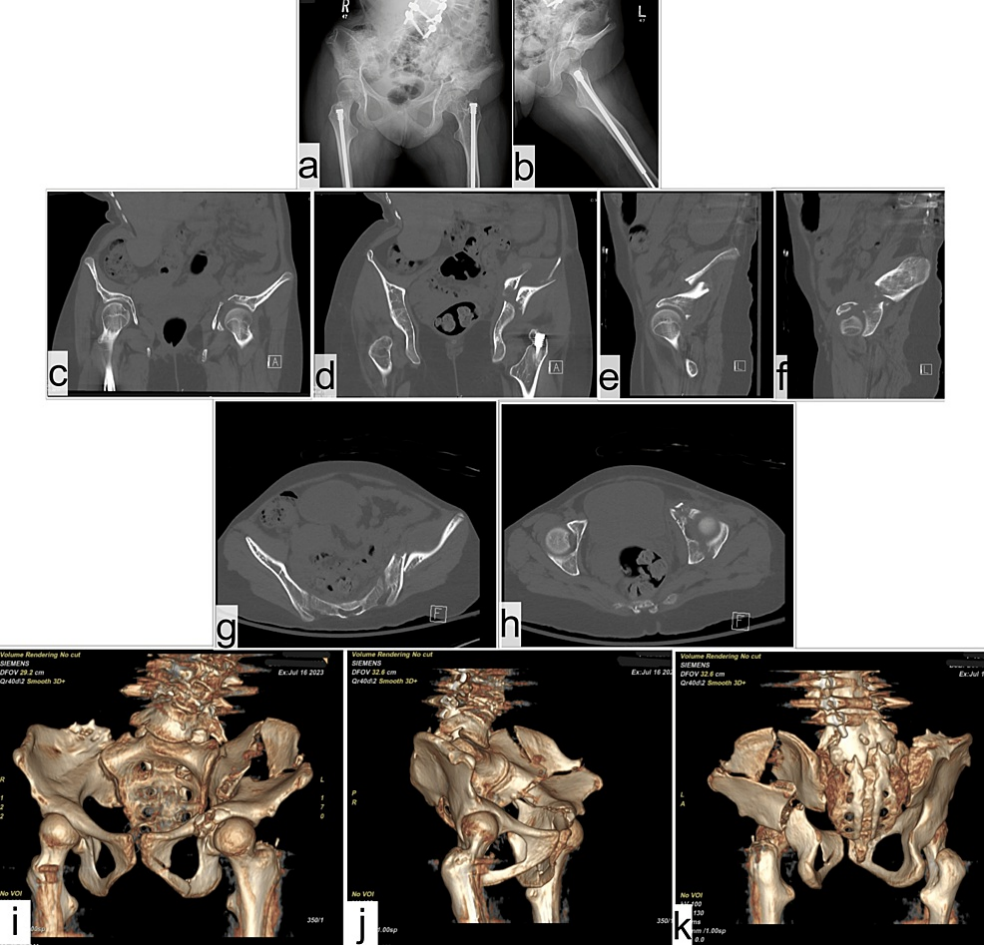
Preoperative imaging showing X-rays (A: AP pelvis, B: lateral left hip), and CT scan (C,D: coronal) (E,F: sagittal), (G,H: axial), (I,J,K: 3D reconstruction) showing both column associated fracture of left acetabulum with involvement of the iliac wing. AP: anterior-posterior
